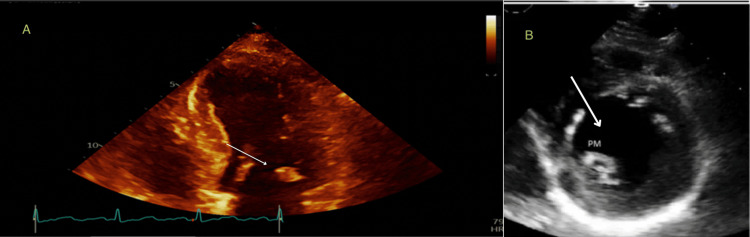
Transthoracic echocardiogram Image A: The arrow showed a small rounded mass at the tip of the posterior mitral leaflet. Image B: Parasternal short axis at the level of the papillary muscle. The arrow showed a single posteromedial papillary muscle. PM: Posteromedial
